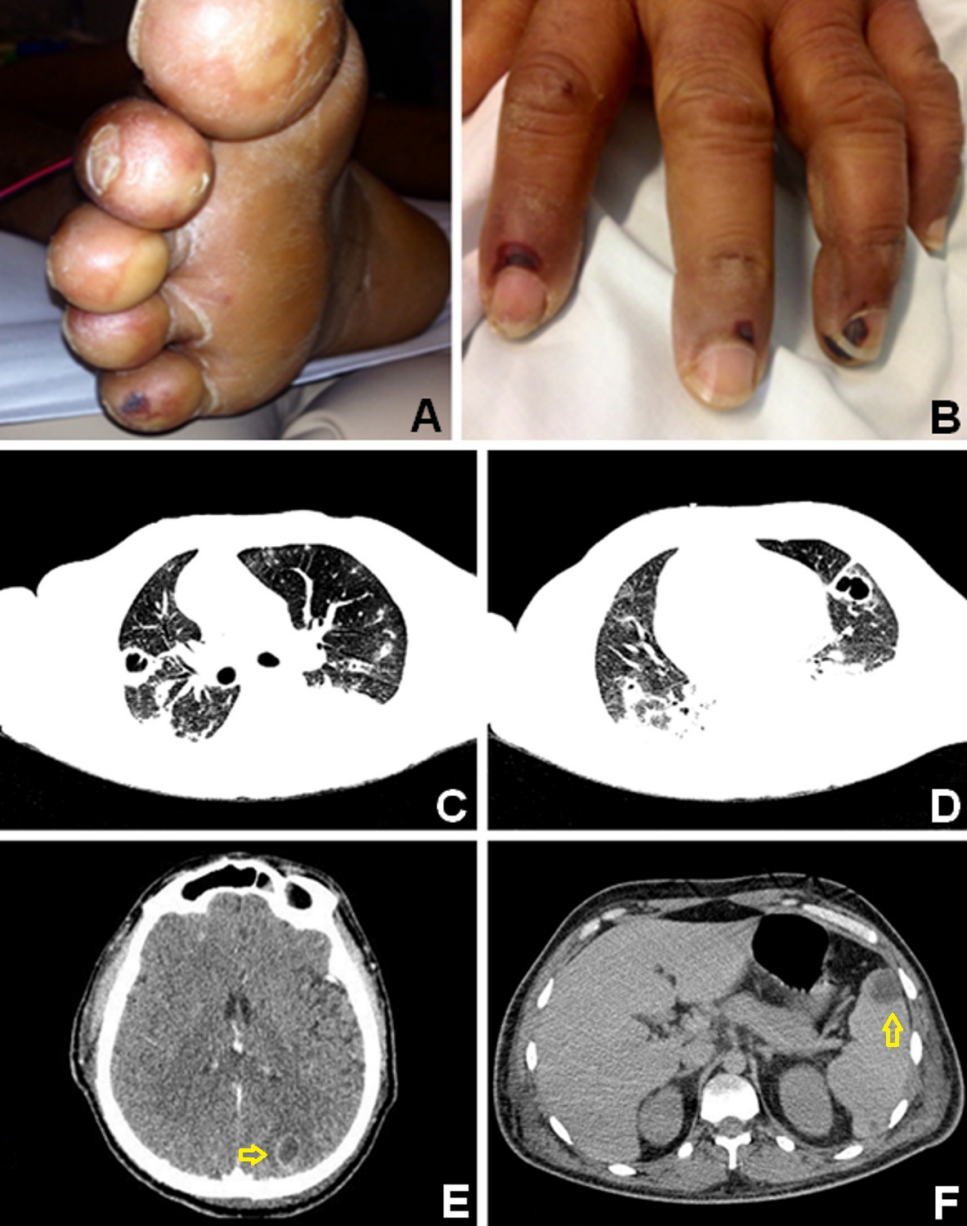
Evidence of peripheral (A and B) embolization from the aortic valve vegetation. Computed tomography scans show bilateral pulmonary (C and D), as well as cerebral (E) and splenic (F) embolisms (arrows).
